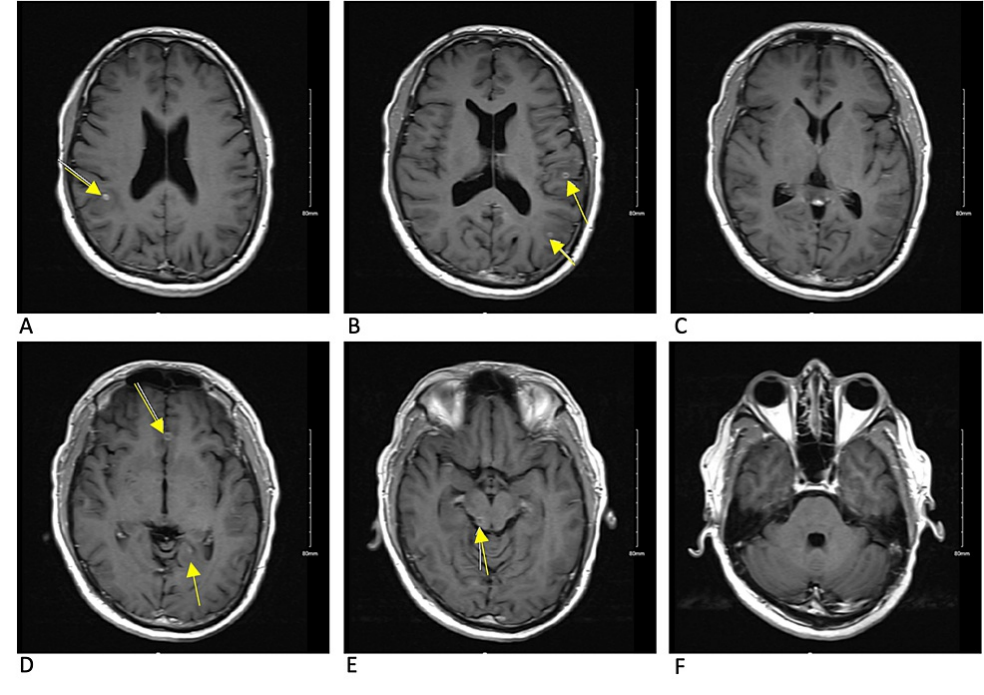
MRI brain T1 axial post-contrast sequence five days after initiation of dual antiparasitic therapy An overall decrease in the size of the ring-enhancing lesions is consistent with early response to antiparasitic treatment. Cysts are now progressing through the stages of degeneration and are mostly in the granular-nodular stage. The cysts seen in previous scans in the anterior frontal lobes are resolved (panes A and B). The cysts in the left and right parietal lobes have decreased perilesional edema (panes A and B). The interhemispheric, hippocampal, and right posterior midbrain cysts have decreased in size (panes D and E).
